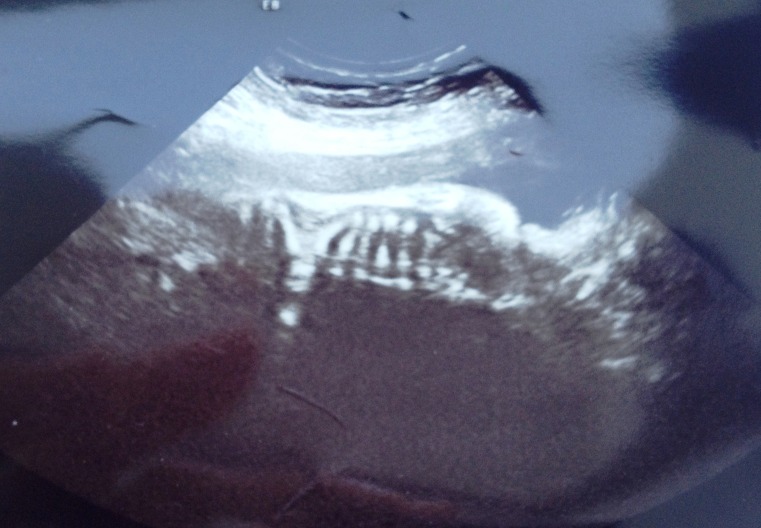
At 18 weeks of gestation – ultrasound aspect in a sagittal section - note thoracic and lumbar hemivertebrae with abnormal alignment of the vertebral bodies and kypho-scoliosis
